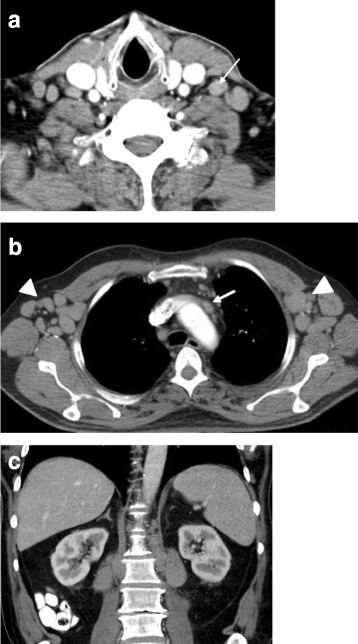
69-year-old woman at the time of diagnosis with CLL. a Axial CT image of the neck obtained during arterial phase demonstrates bilaterally enlarged supraclavicular lymph nodes (thin white arrow). b Axial CT images of the chest obtained during arterial phase show multiple enlarged bilateral axillary lymph nodes (arrowheads) and mildly prominent prevascular lymph nodes (white arrow). c Coronal reconstructed CT image of the abdomen shows mild splenomegaly
